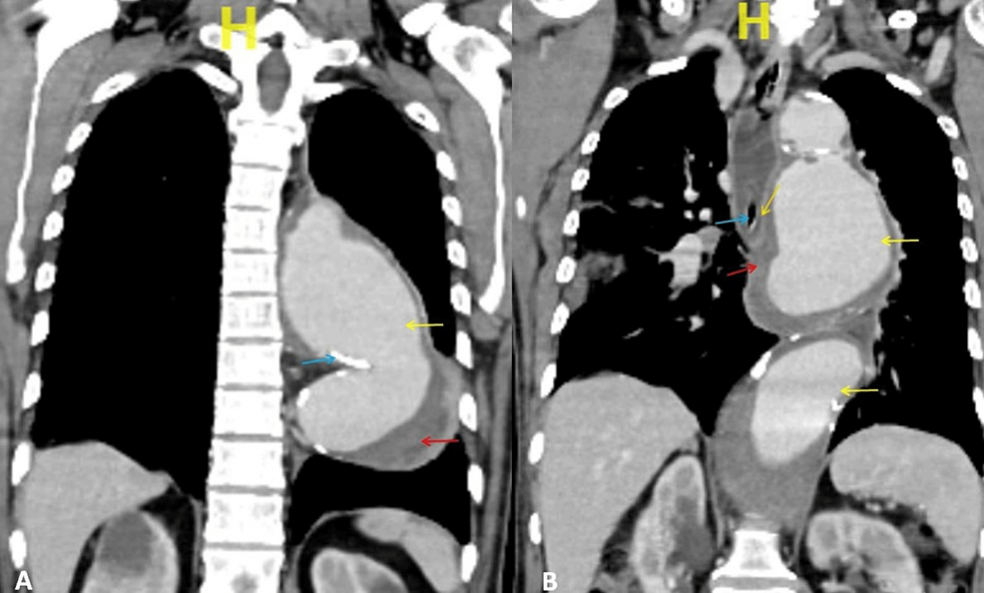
Computed tomography (coronal view) A: Descending aortic aneurysm (yellow arrow), thrombus within the aneurysm (red arrow), and atherosclerotic calcification within the wall of the aneurysm (blue arrow), and B: Descending aortic aneurysm (yellow arrow), thrombus within the aneurysm (red arrow), nasogastric tube within the esophagus (blue arrow), and the probable site of aortoesophageal fistula (orange arrow).
